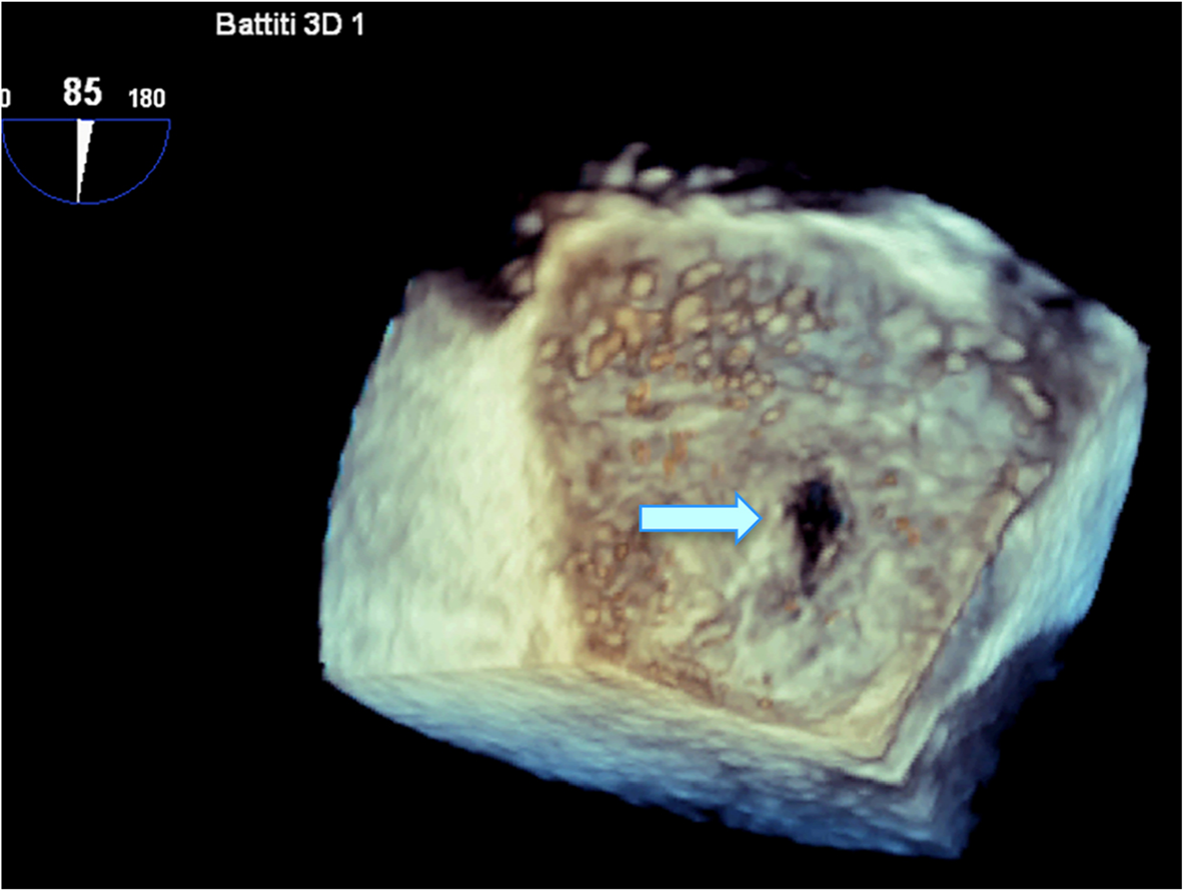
Transesophageal 3D echocardiographic view showing the patent foramen ovale (*light blue arrow*)
